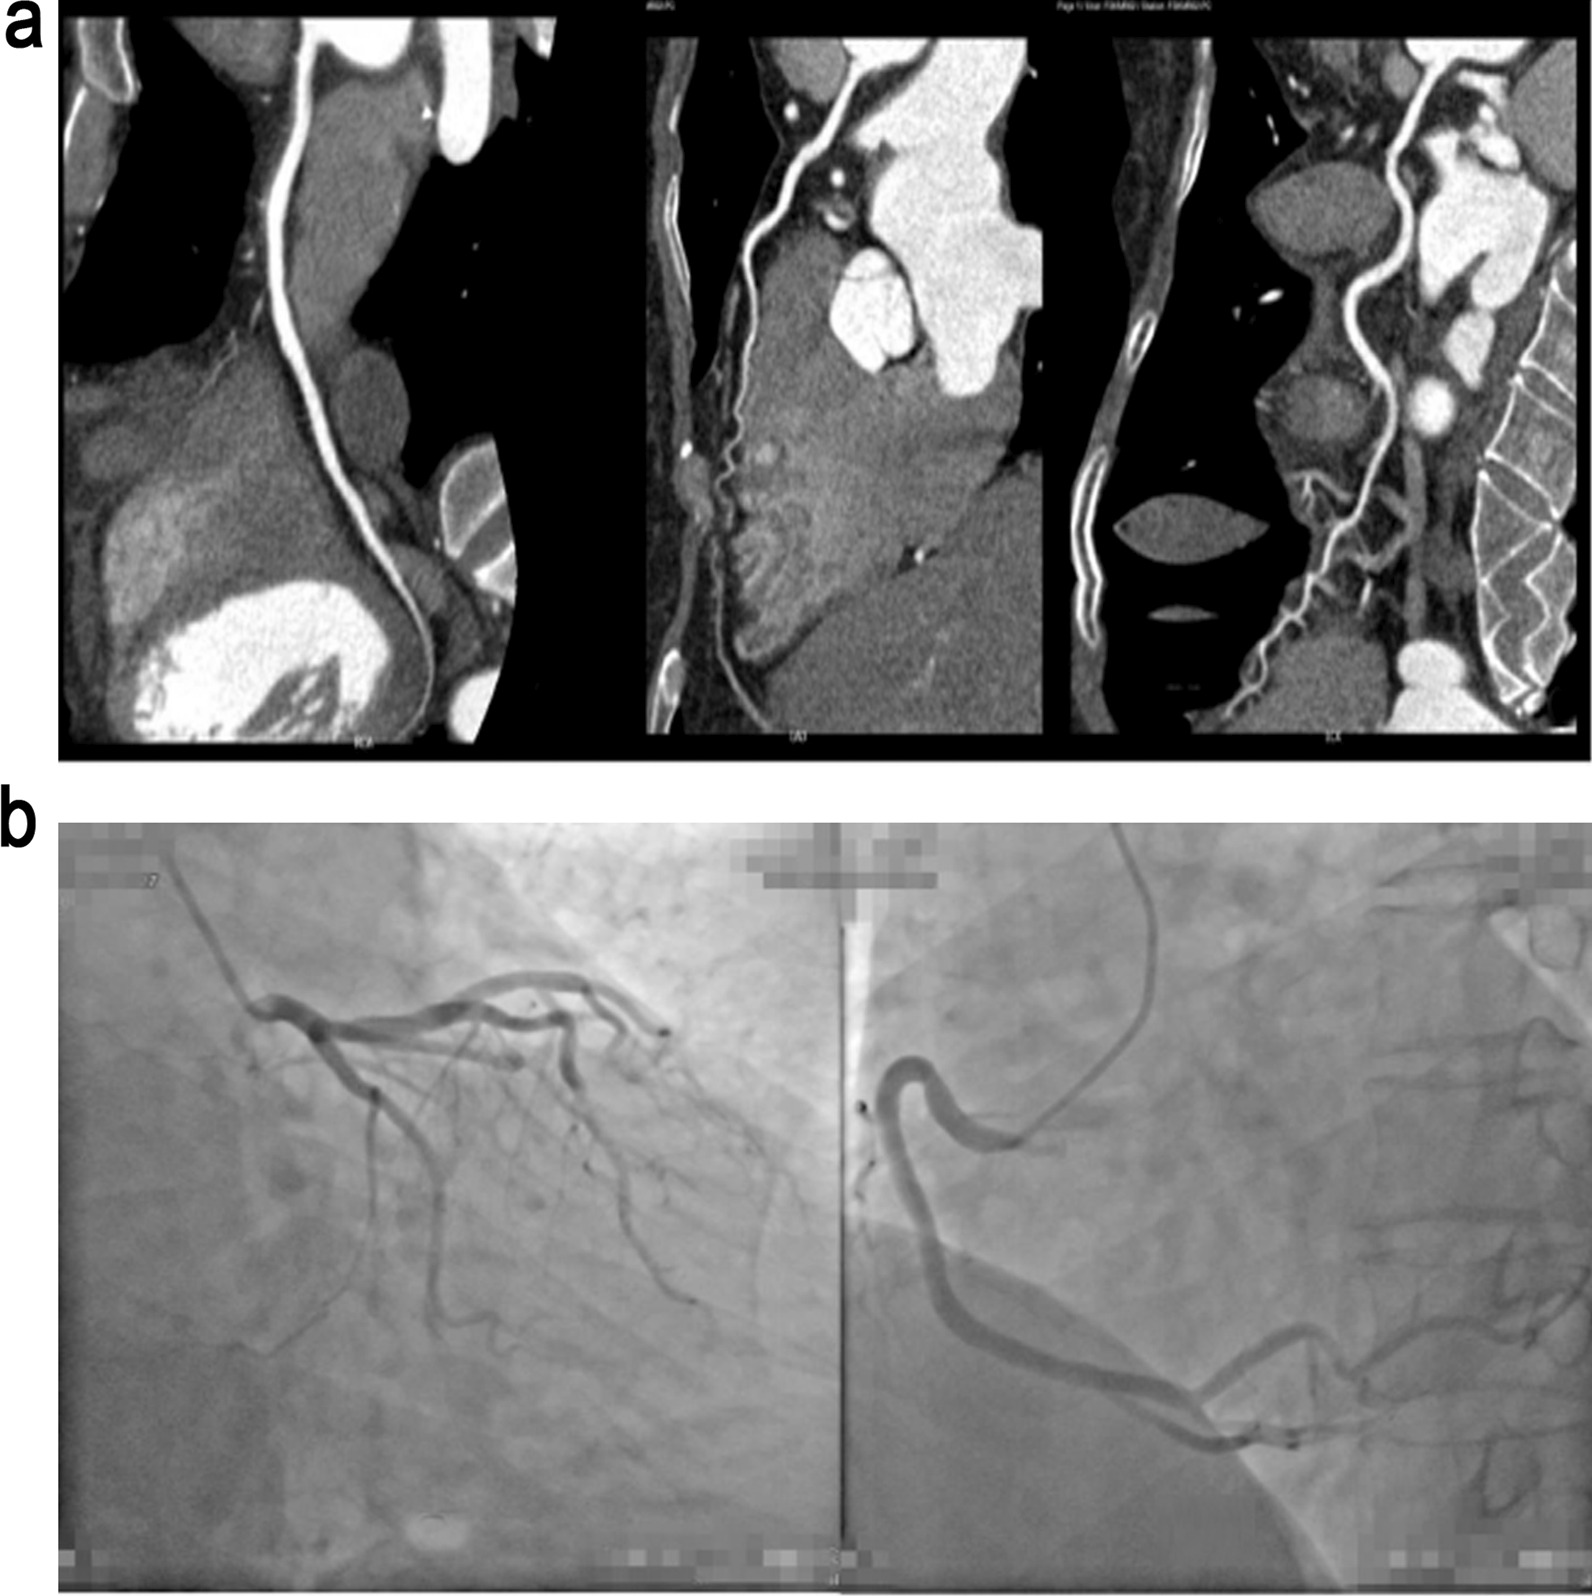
Representative images of coronary computed tomographic angiography (CTA) and coronary angiography (CAG) in two cases. a CTA images indicated that no obvious stenosis was observed in coronary arteries (RCA, LAD, and LCX) in a 48-year-old female patient with MINOCA. b CAG images indicated that mild stenosis (less than 50%) was observed in RCA coronary artery in a 74-year-old male patient with MINOCA
